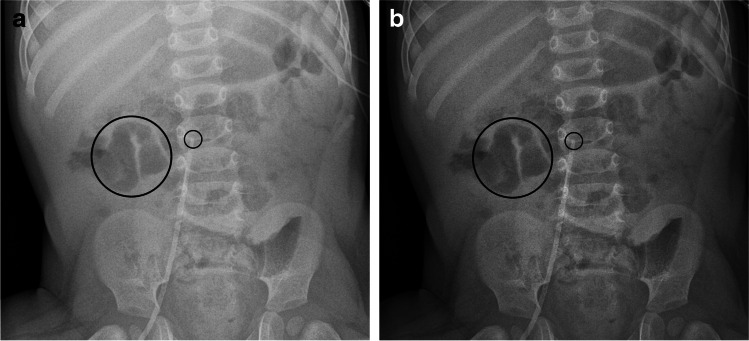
An anteroposterior abdominal radiograph (59.8 kV, 2.0 mAs, 0.57 cGy·cm^2^), unprocessed image (a) versus a SimGrid image (b) in a 1-year-old boy with central venous catheter from right femoral vein with the tip in the inferior vena cava (*small black ring*) and intestinal gas in the small bowel (*large black ring*)
